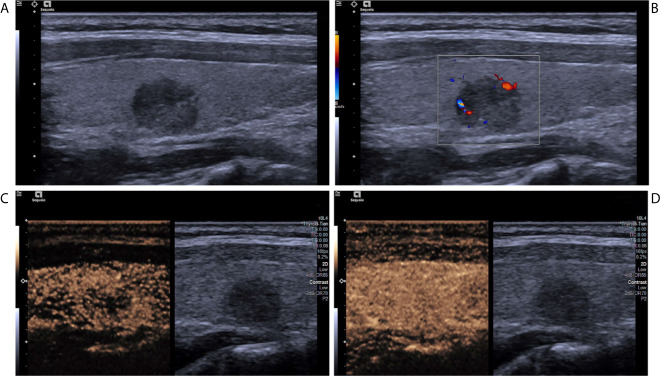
A papillary thyroid carcinoma in a 25-year-old girl. (A) Greyscale ultrasound showed that there was a solid hypoechoic nodule in the right lobe of the thyroid, with irregular margin, wider-than-tall shape, and punctate echogenic foci. The nodule was ACR TI-RADS category 5. (B) Color Doppler showed peripheral vascularity. (C) Contrast-enhanced ultrasound revealed the trend that contrast agent enters the nodule from the periphery to the center (centripetal enhancement) at the time of the 14th second after the injection of contrast agent. (D) Contrast-enhanced ultrasound revealed iso-enhancement at peak (the 22th second after the injection of contrast agent), with ill-defined enhanced border and absence of a regular enhanced ring.
